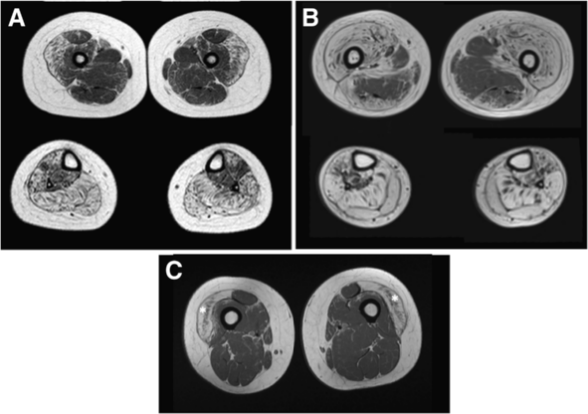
Muscle MRI (coronal images: thighs (*up*); calves (*down*); T1-weighted sequence). Diffuse bright appearance of the anterior compartment of the thighs, particularly in the vastus lateralis muscles, and the posterior compartment of the calves in individual F1 at 7 years of age (a); more severe stage with a relative sparing of the posterior compartment of the thighs in proband F2 at 30 years (b). Specific involvement of the vastus lateralis muscles (*asterisks*) with sparing of other thigh muscles in individual F9 (c)
